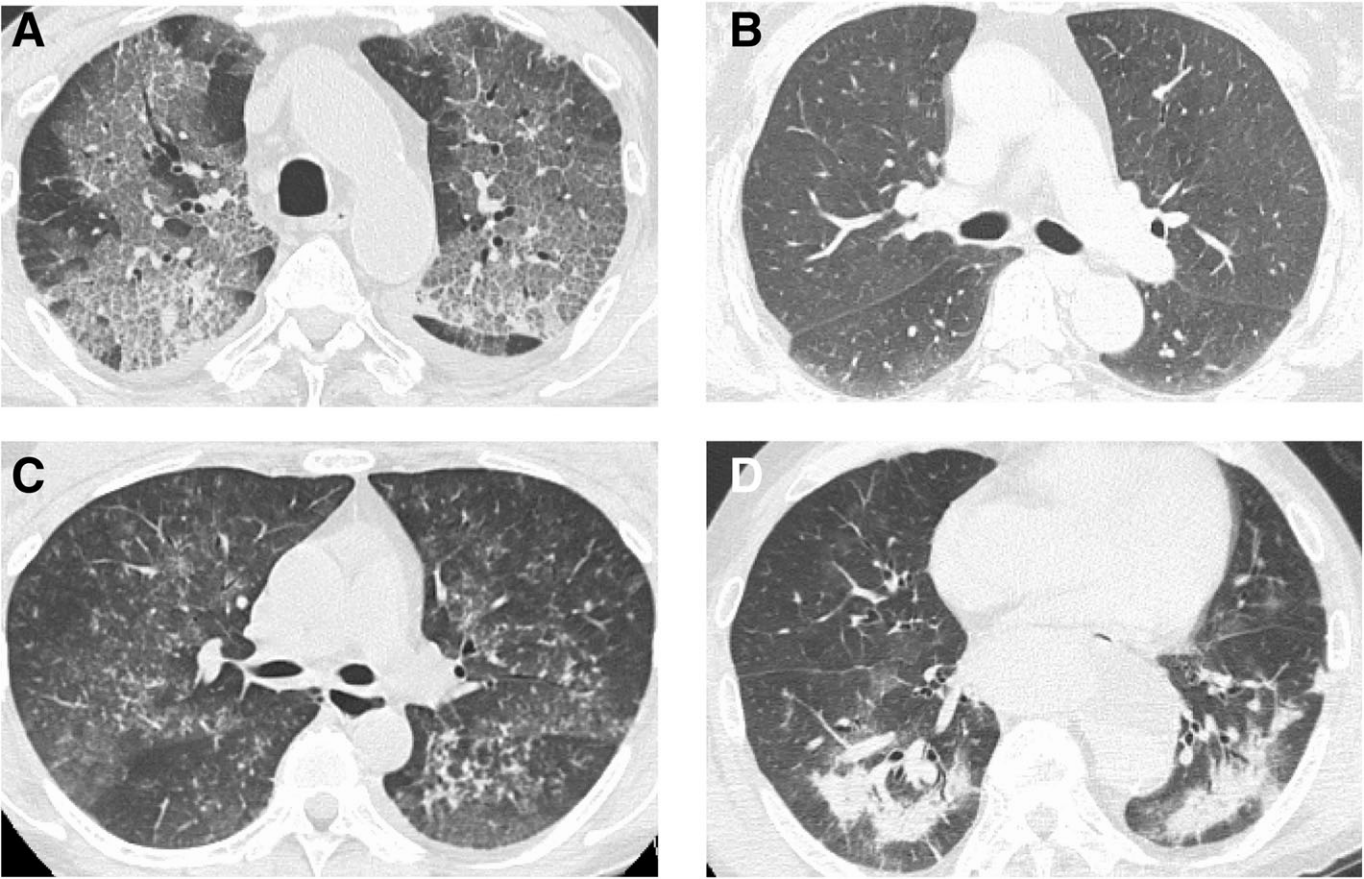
Axial high resolution CT slices of the chest in the lung window of representative four non-HIV cases with proven PjP show crazy paving GGO (a), GGO without crazy paving appearance (b), centrilobular nodules (c), and consolidations (d)
